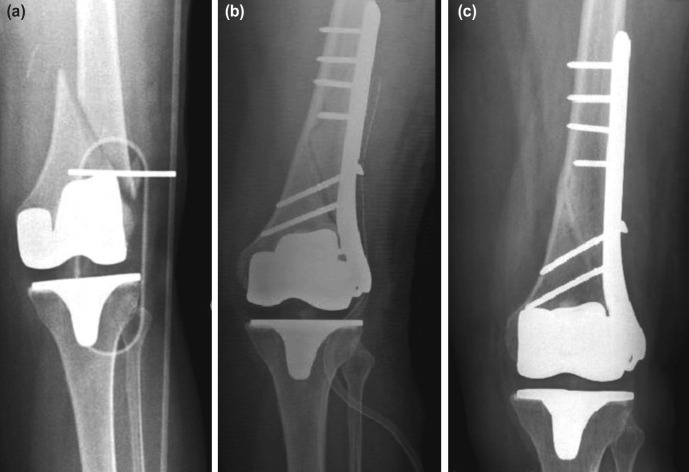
(a) Lewis Rorabeck Type 2 supracondylar femoral periprosthetic fracture. (b) Post-operative first day radiography. (c) 16th week radiography.
